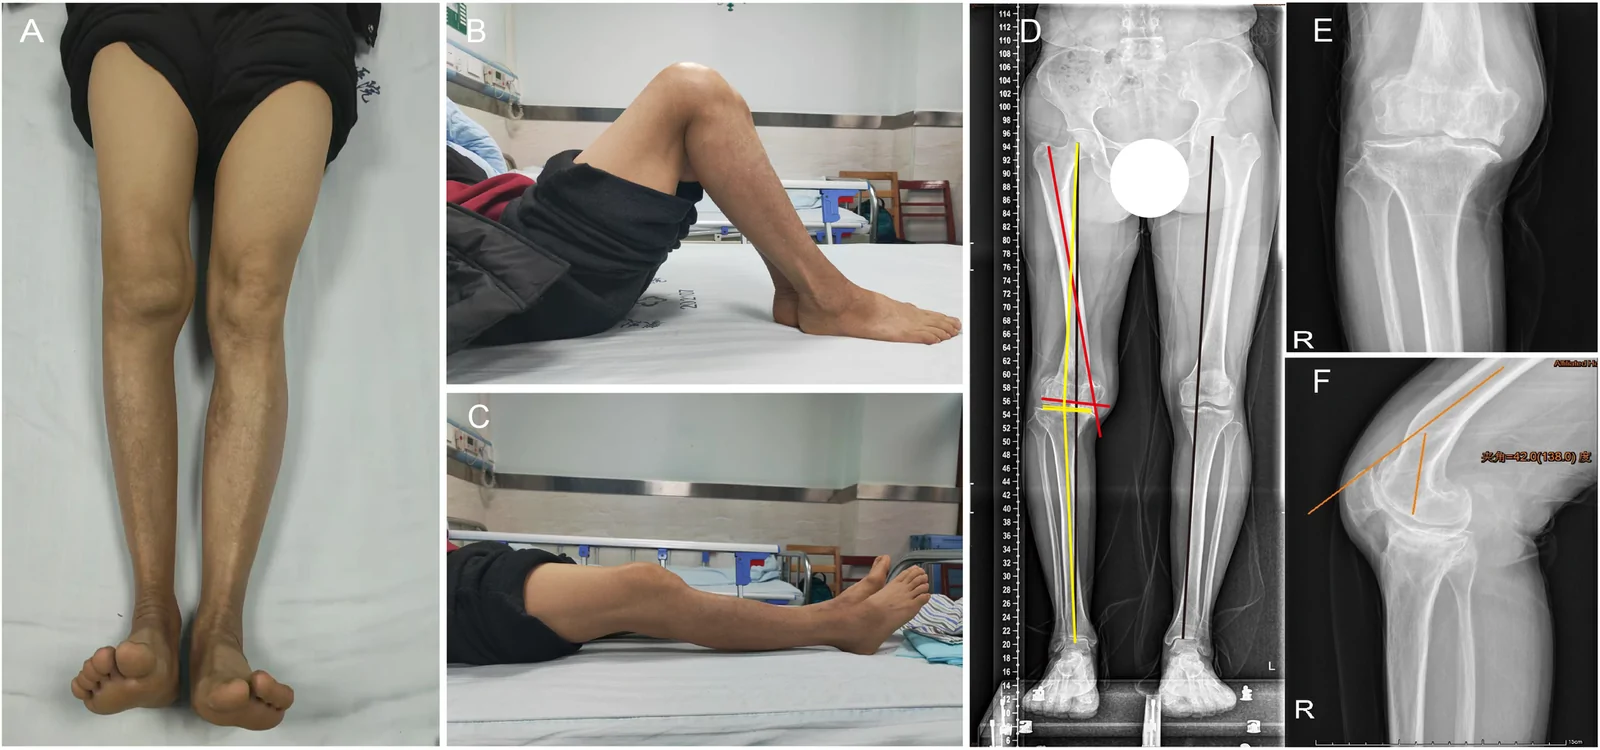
Preoperative clinical appearance and radiographic deformity analysis (A) clinical photograph showing overall varus alignment of both lower limbs. (B) Active knee flexion was limited to 110°. (C) Active extension showed an approximately 20° flexion contracture. (D) Standing full-length radiograph showing global varus alignment, with an HKA of 174.4° (5.6° varus) and a MAD of 20 mm medial to the knee center, despite local distal femoral valgus. The red and yellow arcs indicate the aLDFA (74.3°) and aMPTA (82.7°), respectively; the black line indicates the lower-limb mechanical axis. (E) Anteroposterior knee radiograph showing end-stage osteoarthritis and local distal femoral metaphyseal valgus. (F) Lateral radiograph showing severe anterior bowing of the distal femur; the deformity apex was localized approximately 67 mm proximal to the joint line on CT.
